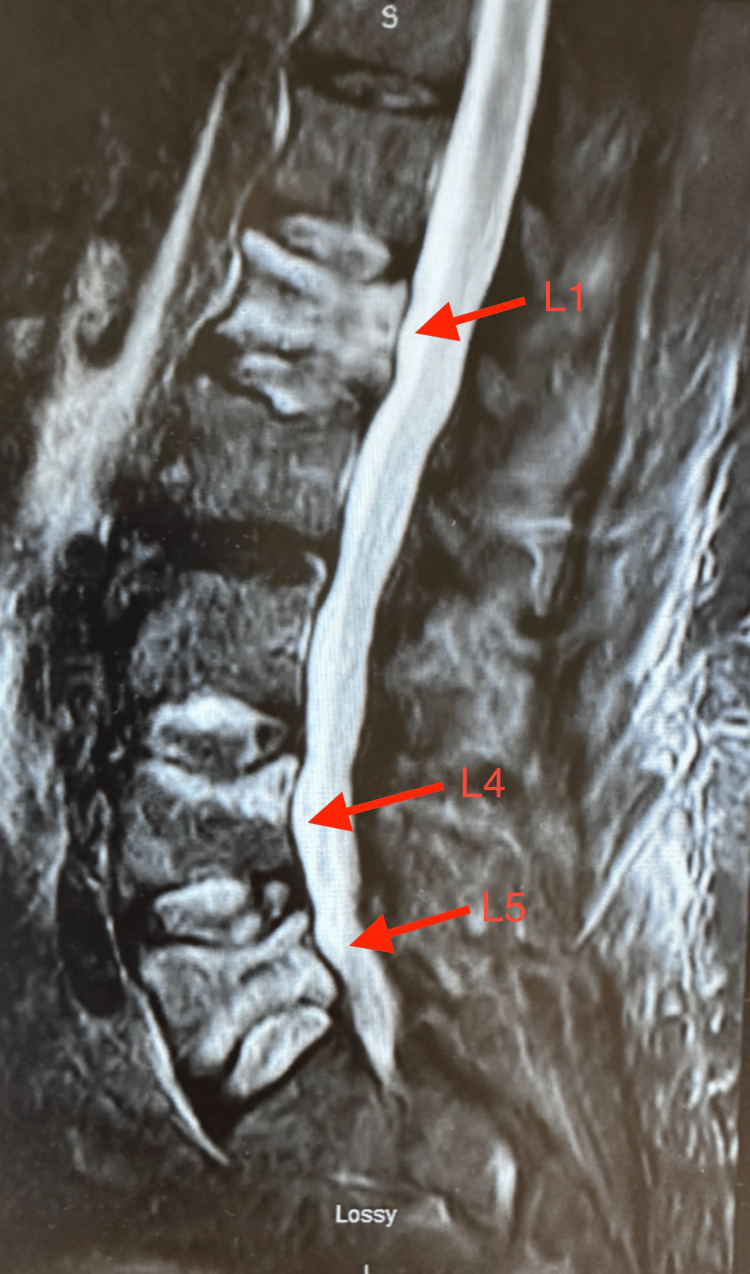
Sagittal T2-weighted MRI of the lumbar spine demonstrating hyperintense marrow edema at L1, L4, and L5 (red arrows). Vertebral bodies are consistent with acute to subacute compression fractures. Image captured using a smartphone camera due to limitations in accessing downloadable imaging files. All identifiable information was removed.
